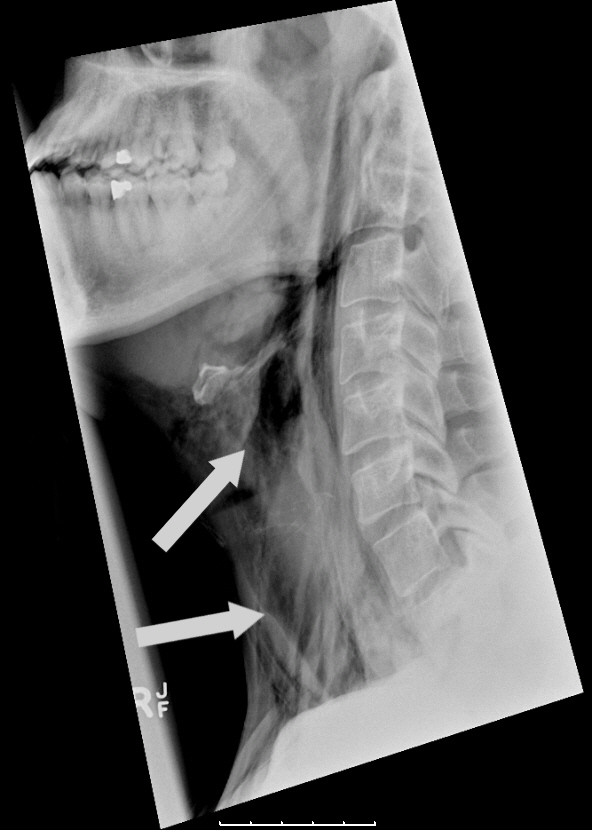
Lateral X-ray of neck showing subcutaneous emphysema.
